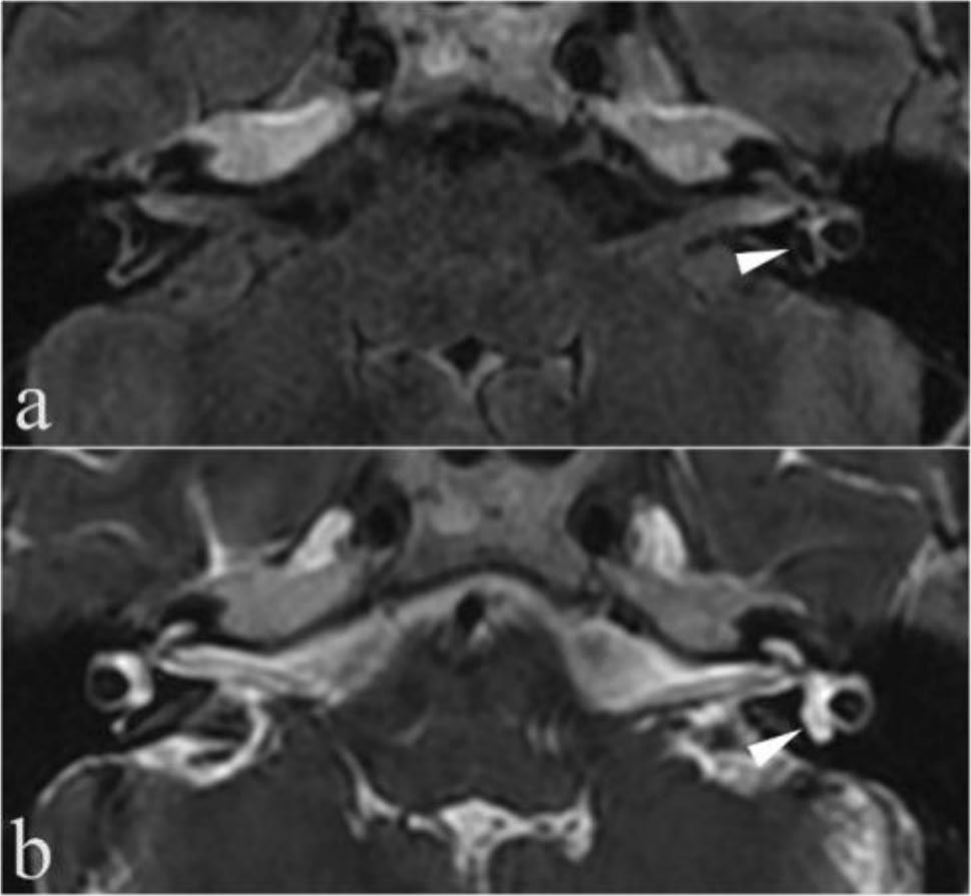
A 62-year-old woman with left Ménière’s disease and posterior semicircular canal dysplasia (a, b). 3D-FLAIR (a) and 3D-T2WI (b) showed left posterior semicircular canal dysplasia (arrowheads). The endolymph compartment occupies more than 50% of the short and enlarged posterior semicircular canal.
